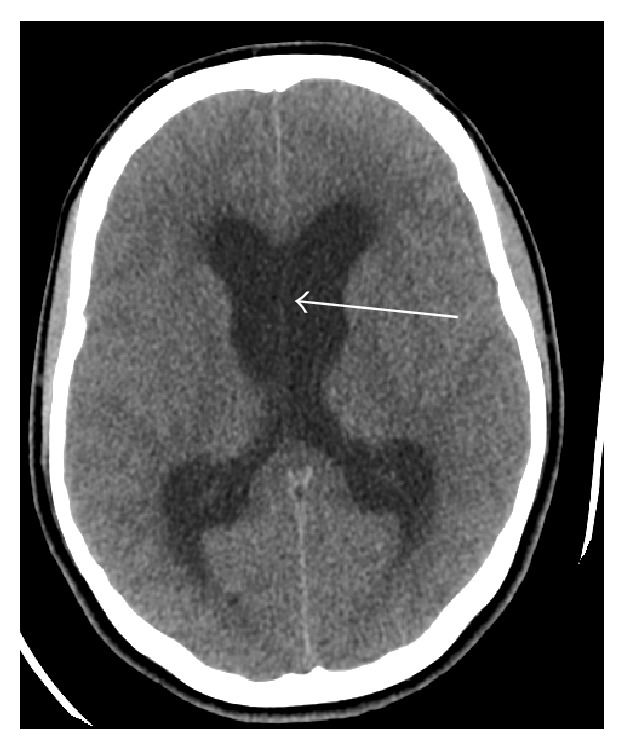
Plain axial CT scan of brain-showing severe obstructive lateral ventricle hydrocephalus shown by white arrow with signs of brain edema.
